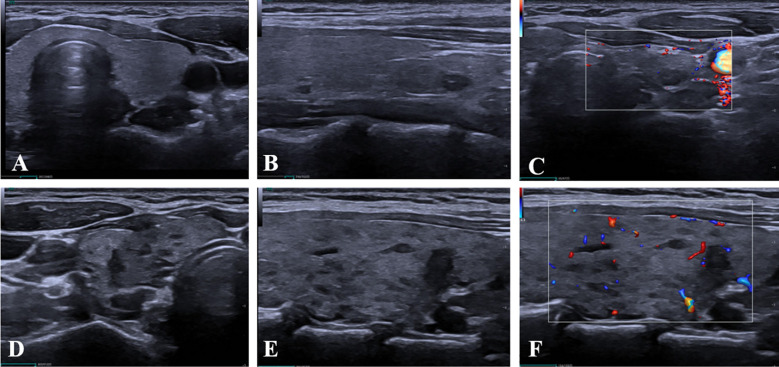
Representative ultrasound criteria for the diagnosis of Hashimoto’s thyroiditis (HT) in this study. (A–C) Thyroid nodules without coexisting HT. Gray-scale ultrasound images of the transverse (A) and longitudinal (B) planes demonstrate a homogeneous parenchymal echotexture. (C) Color Doppler imaging reveals minimal intraparenchymal vascularity. (D–F) Thyroid nodules with coexisting HT. Gray-scale ultrasound images of the transverse (D) and longitudinal (E) planes show an inhomogeneous echotexture with diffusely decreased echogenicity and patchy hypoechoic areas. (F) Color Doppler imaging demonstrates a moderate degree of intraparenchymal vascular signals.
